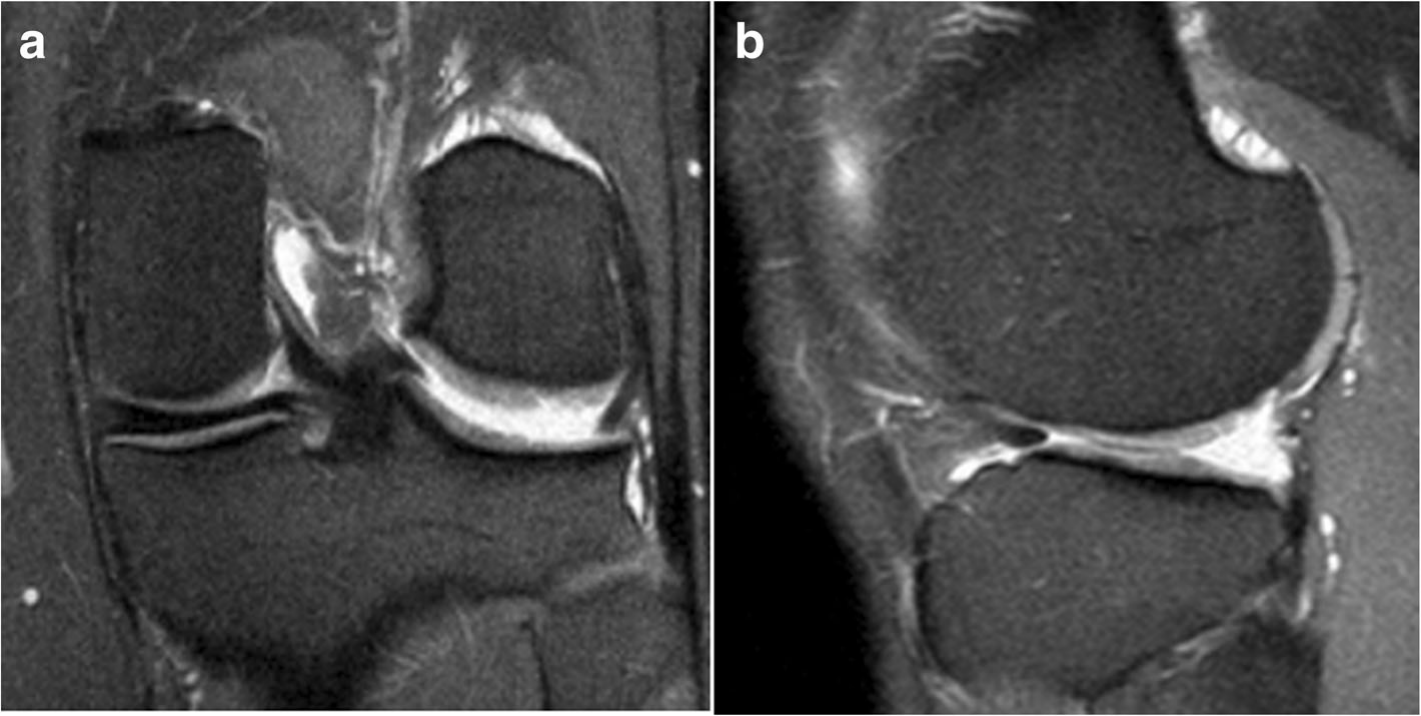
A recent frontal (a) and sagittal (b) MRI Patient 2
